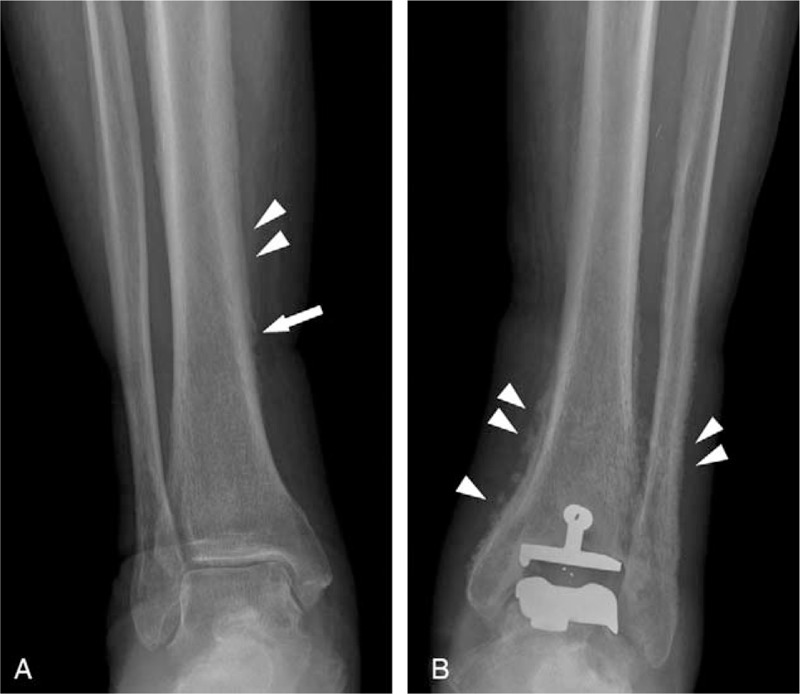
(A) Frontal radiograph of the right lower leg demonstrates a single lamellar periosteal reaction at the tibial shaft (arrowheads). A focal irregular periosteal reaction is also noted (arrow). (B) Frontal radiograph of the left lower leg shows spiculated and thick irregular periosteal reactions at the distal tibia and fibula. Note total ankle arthroplasty state at the left ankle (arrowheads).
